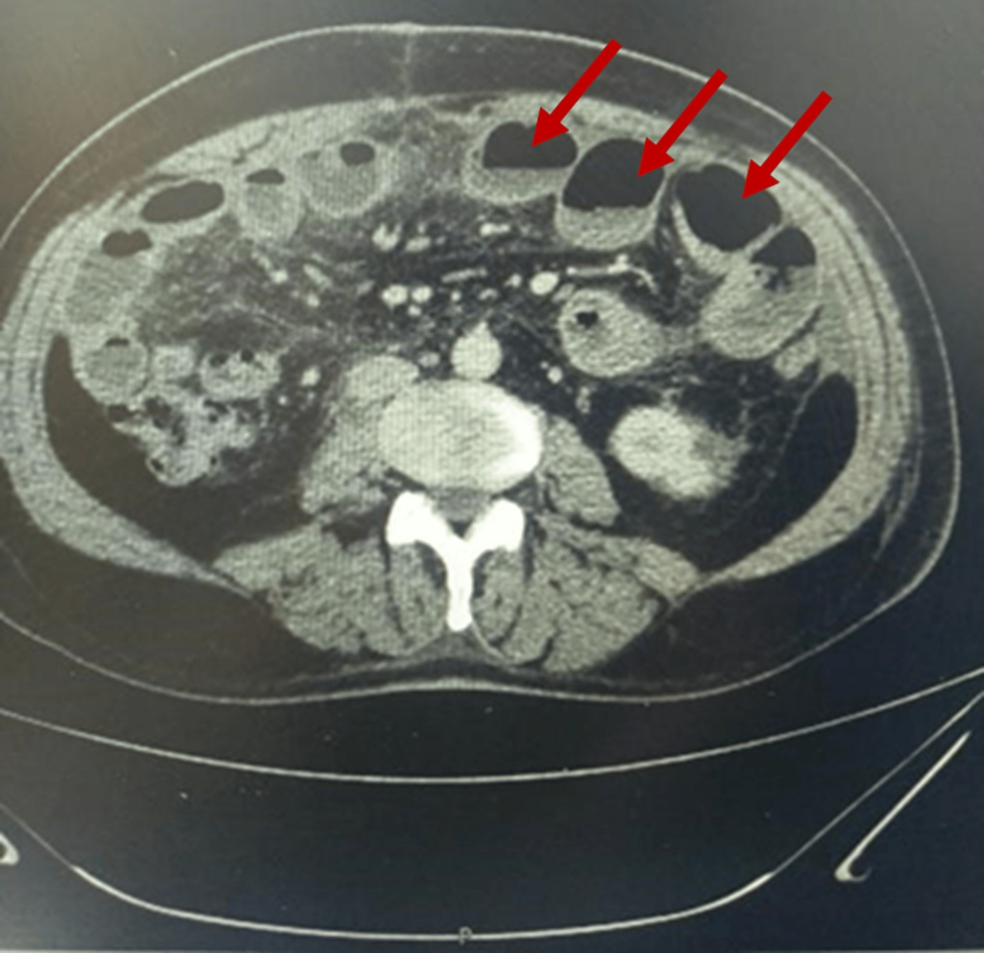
An axial cut of an abdominal CT scan showed small bowel dilatation with air-fluid levels (red arrows) and loops proximal to collapsed loops.
